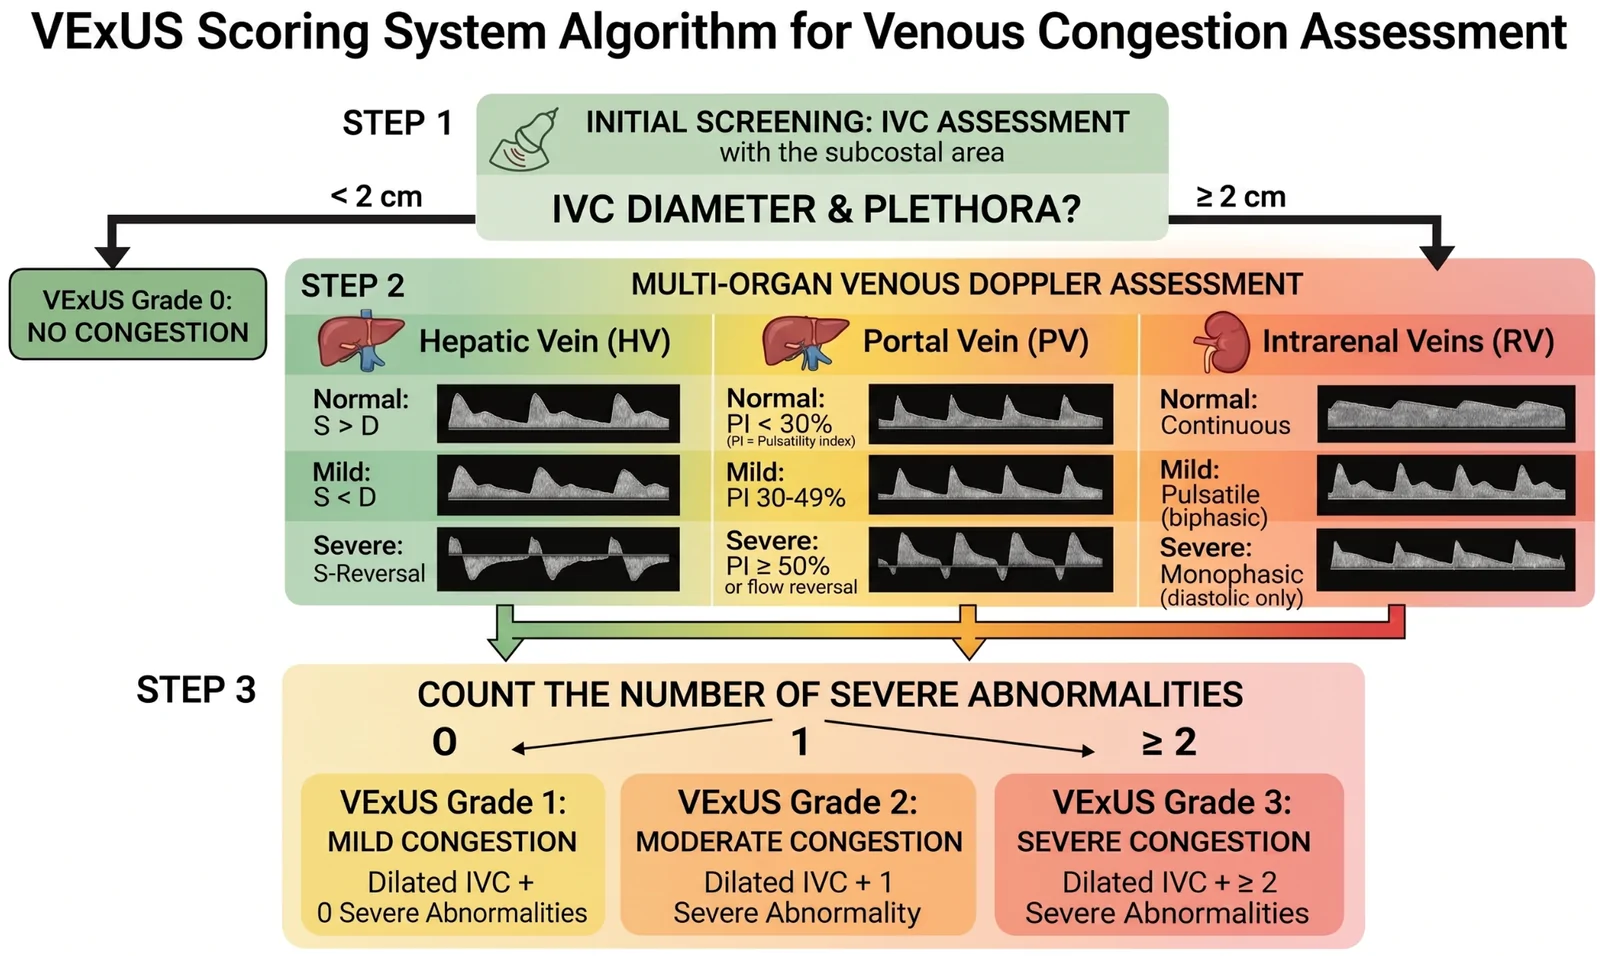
VExUS (Venous Excess Ultrasound) scoring system algorithm for venous congestion assessment. The algorithm consists of three sequential steps. Step 1 involves initial evaluation of the inferior vena cava (IVC) diameter; an IVC < 2 cm indicates no congestion (VExUS Grade 0), whereas an IVC ≥ 2 cm requires further venous Doppler evaluation. Step 2 entails multi-organ venous Doppler assessment of the hepatic vein, portal vein, and intrarenal veins. Step 3 determines the final VExUS grade according to the number of severe Doppler abnormalities. Abbreviations: IVC, inferior vena cava; HV, hepatic vein; PV, portal vein; RV, renal vein.
